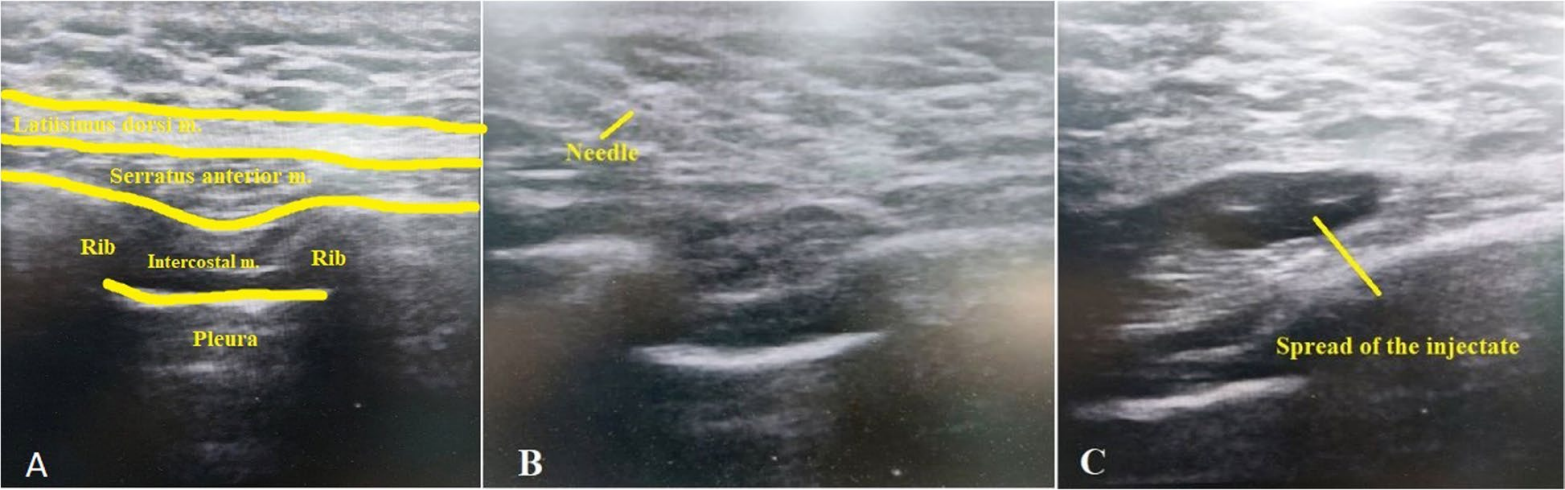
The serratus anterior block technique: (A) Sonographic anatomy of the layers encountered during serratus block. (B) Directing the needle to the plane between serratus and latissimus dorsi muscles. (C) Spread of the local anesthetic injectate in the proper plane
